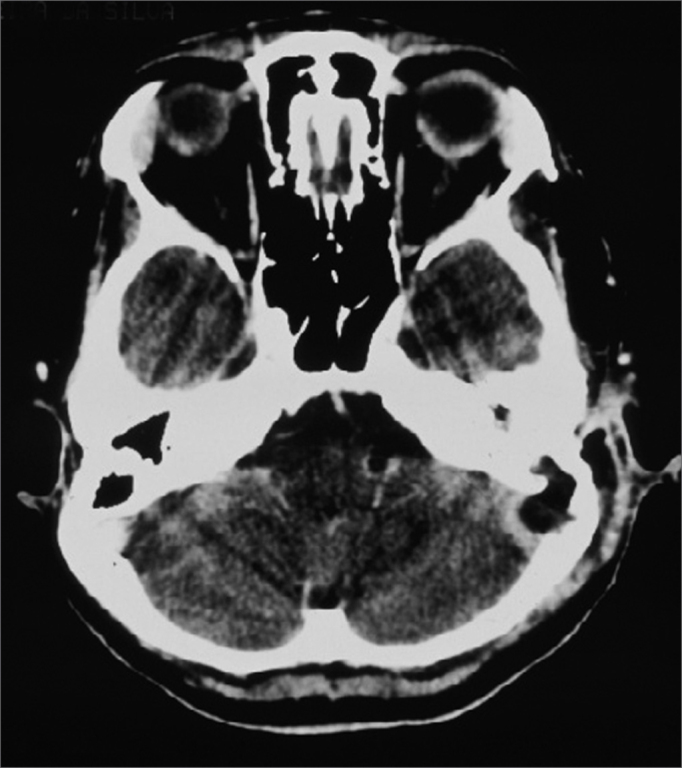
Skull CT scan with contrast, showing contrast highlight around the sigmoid sinus and lack of this highlight inside, with bone erosion to the posterior fossa.
